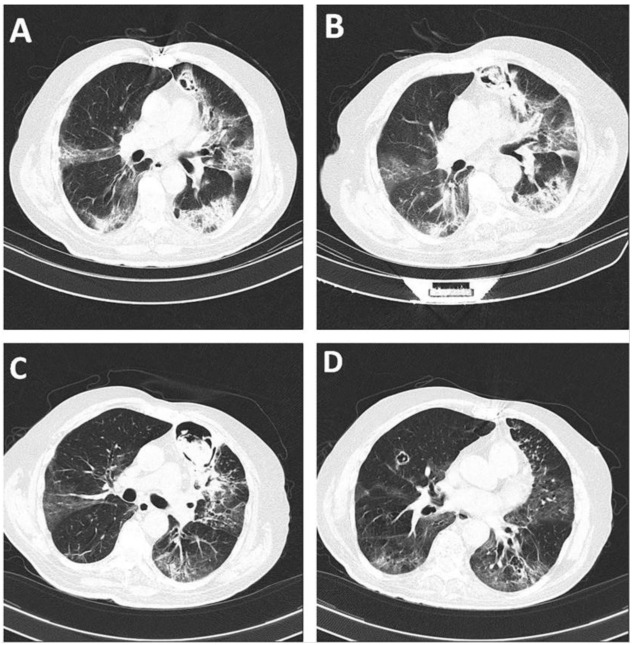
A) Chest computed tomography (CT) scan on the first day of the previous admission that indicates COVID-19 pneumonia and a cavitary lesion measured 20 mm in diameter. B) Chest CT scan on the 3rd week of the previous admission which shows an increase in cavity size. C) Chest CT scan on the second admission which shows a noticeable increase in the size of the previous cavity. D) Chest CT scan on the second admission that shows a new cavity in the opposite lung
